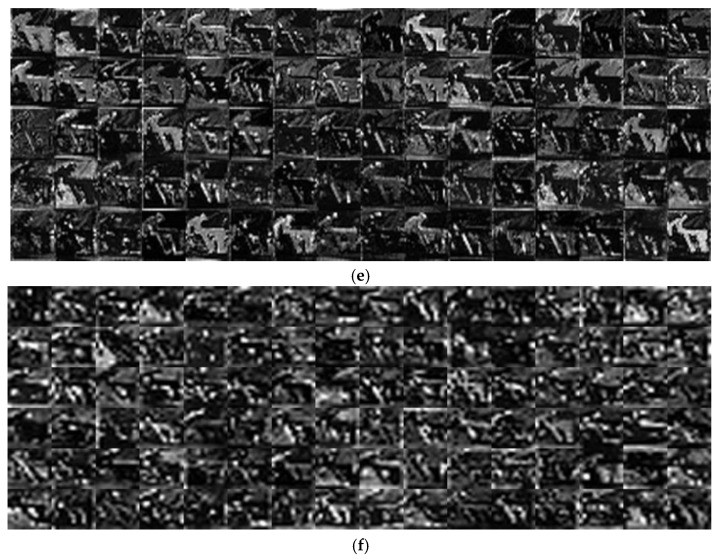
The visualization of the feature map during the process of the proposed model. (a) The original X-ray images of a sample; (b) The visualization of its feature map after the first convolution operation; (c) The visualization of its feature map after the second convolution operation; (d) The visualization of its feature map after the first Dw_Resblock_SE_body module; (e) The visualization of its feature map after the second Dw_Resblock_SE_body module; (f) The visualization of its feature map after the third residual convolution module.
